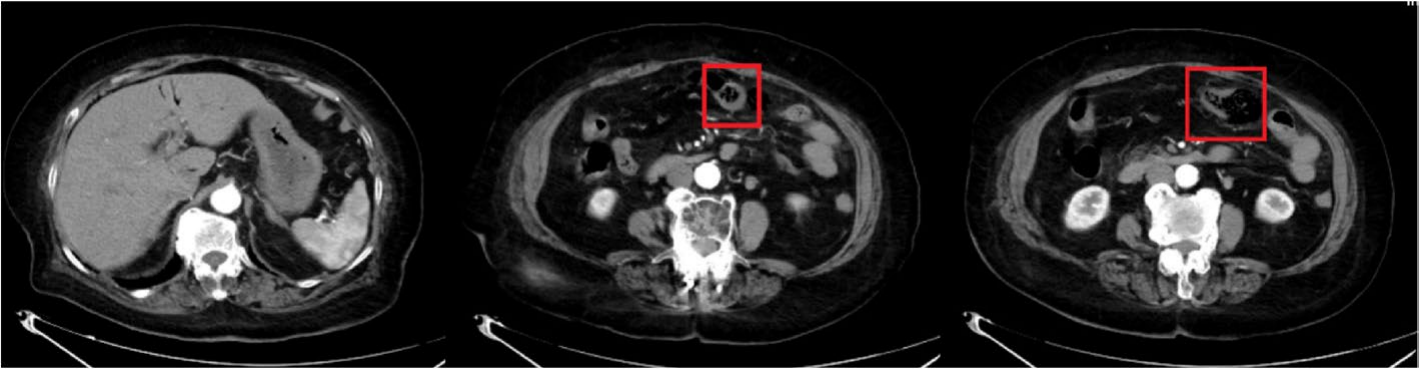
Contrast-enhanced Abdominal CT (External Institution, November 17, 2024)
